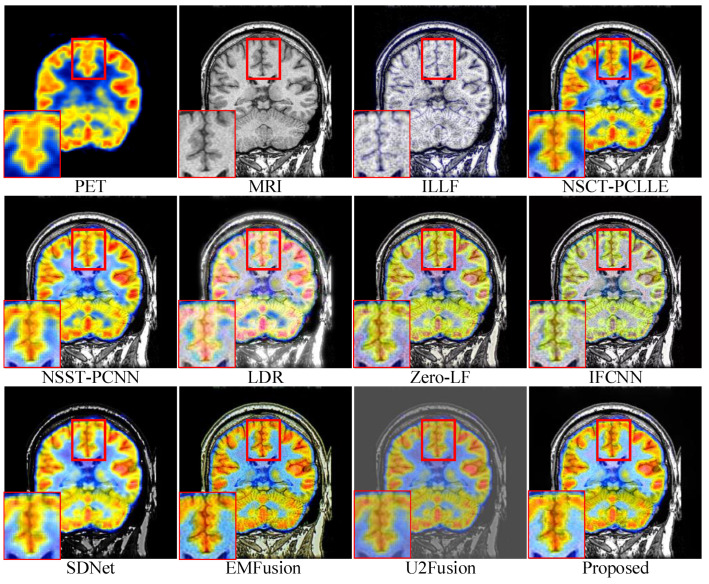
Comparison of performances of various methods on the positron emission tomography (PET)-MRI source images. For a clear comparison, we select a same region (i.e., the red box) in each image and zoom in it in the bottom left corner.
